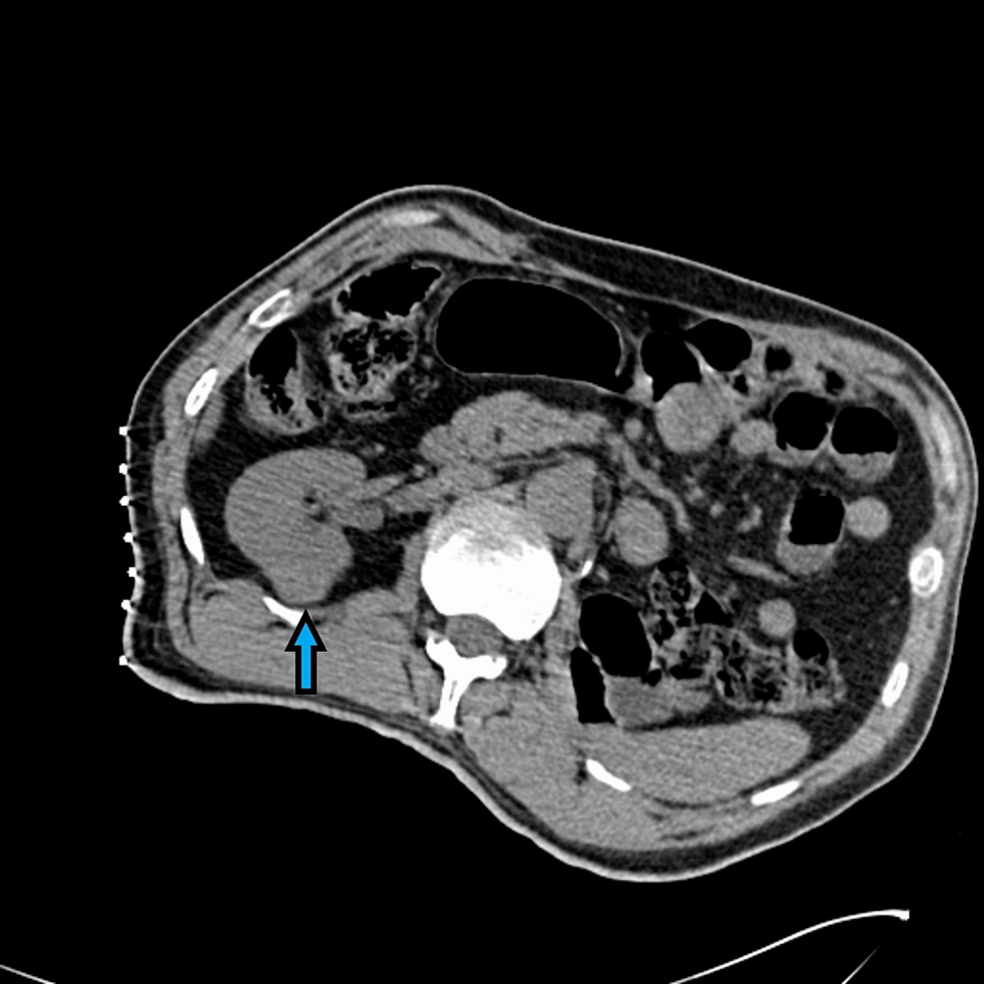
Renal CT demonstrating an exophytic soft tissue mass along the posterior border of the mid pole of the right kidney.
